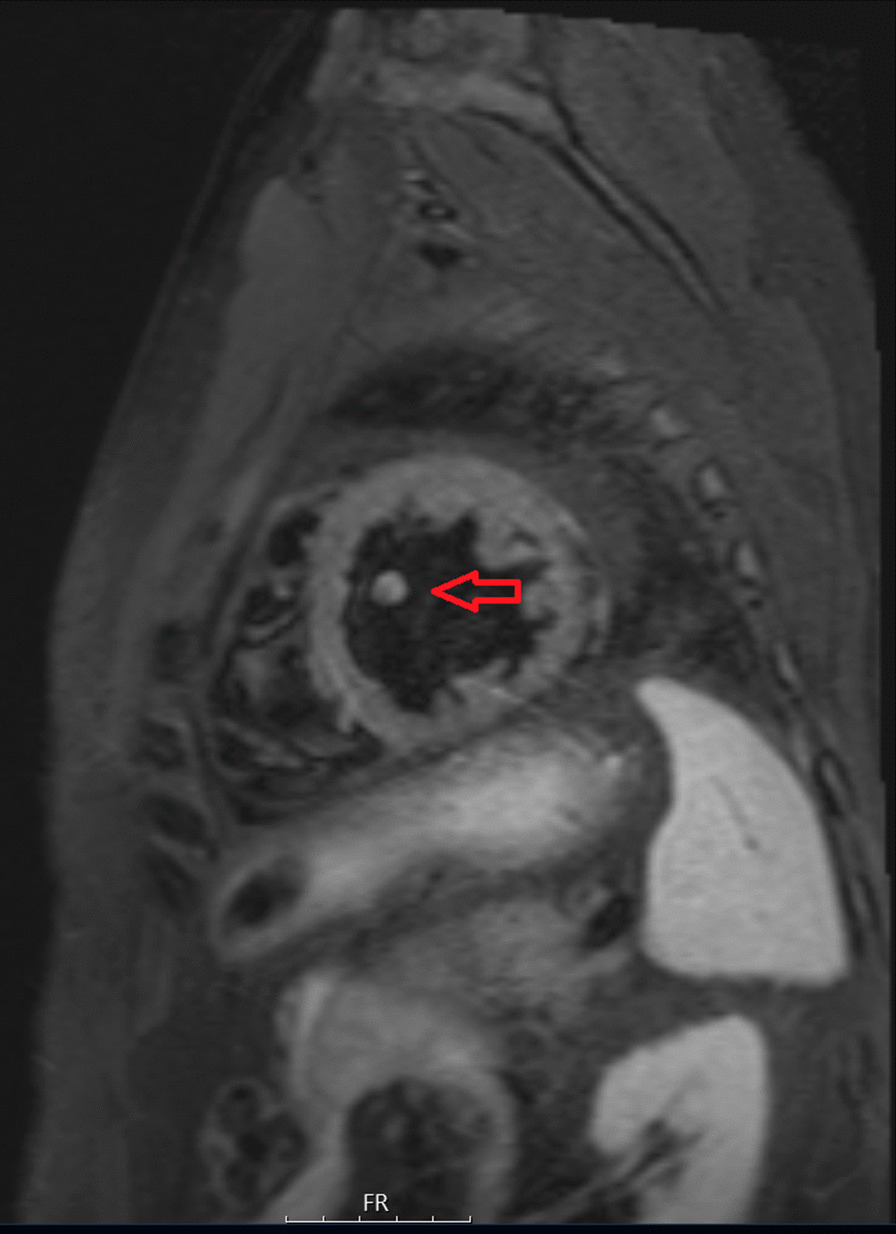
Cardiac magnetic resonance image in short axis view performed on the second day of admission demonstrating a thrombus in the left ventricle
